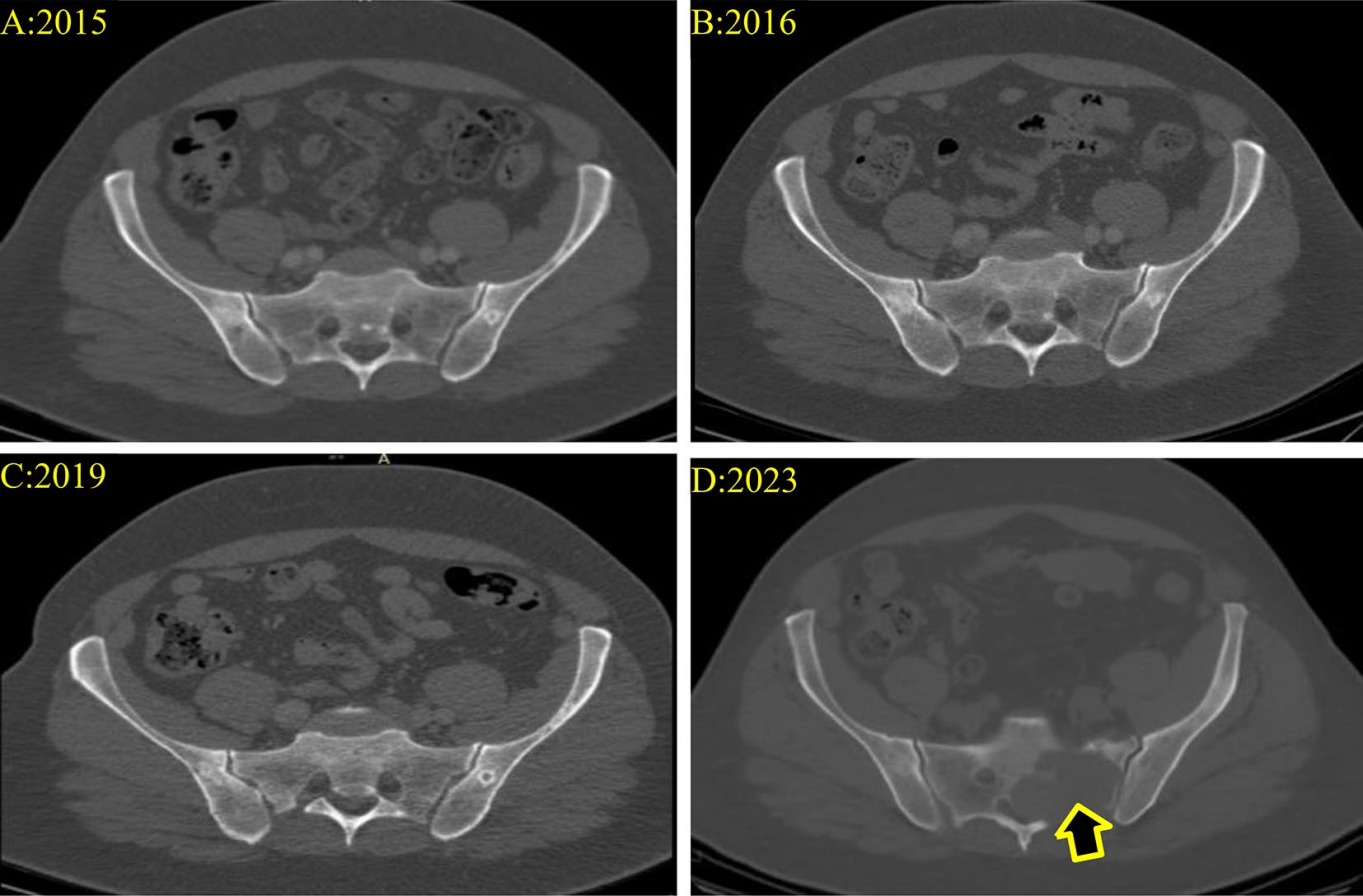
Selected Pelvic CT scans at variable periods interval which reveal normal pelvis CT scan without any suspicious lytic bony lesion (A, B, C). while in 2023 the scan showed an expansile, lobulated osteolytic destructive bony lesion in the left upper sacrum measuring about 5.8 × 6 × 6.2 cm in AP x CC x TS diameters respectively highly suspicious for serious malignant nature (D) (The arrow)
